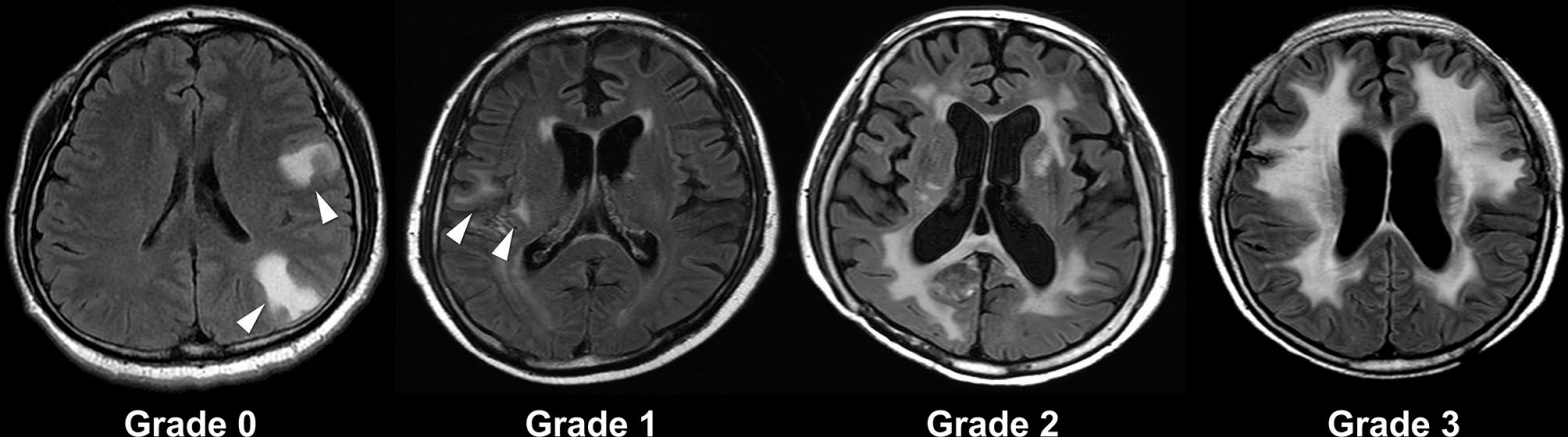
Fluid attenuation inversion recovery (FLAIR) images showing the white matter signal change. Radiation-induced leukoenchepalopathy (RIL) is classified into four grades using the grading system^34^. Tumor-related edema is not regarded as the RIL (white arrowheads).
